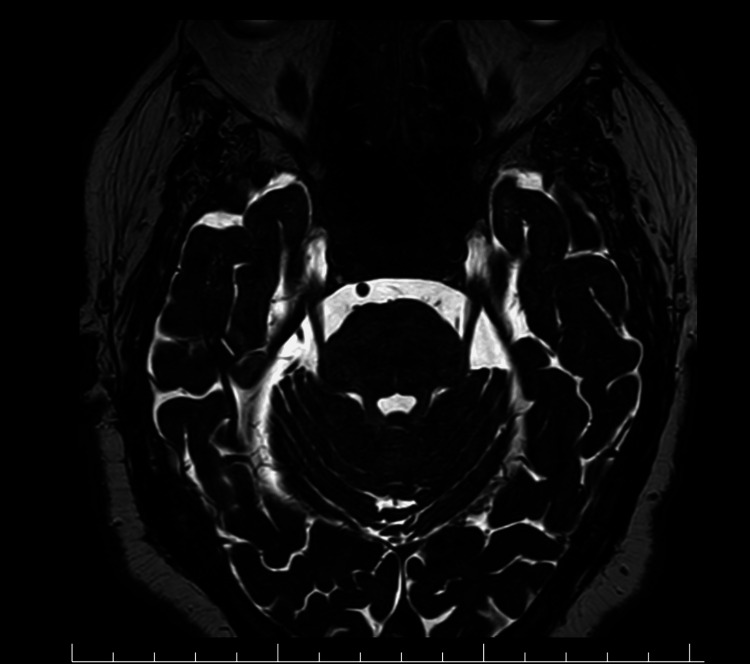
MRI Brain With Contrast Showing No Structural Cause of Third Nerve Palsy Magnetic resonance imaging (MRI) of the brain with contrast demonstrating no evidence of aneurysm, mass lesion, midbrain infarction, or cavernous sinus pathology. No compressive etiology for the left oculomotor nerve palsy is identified.
